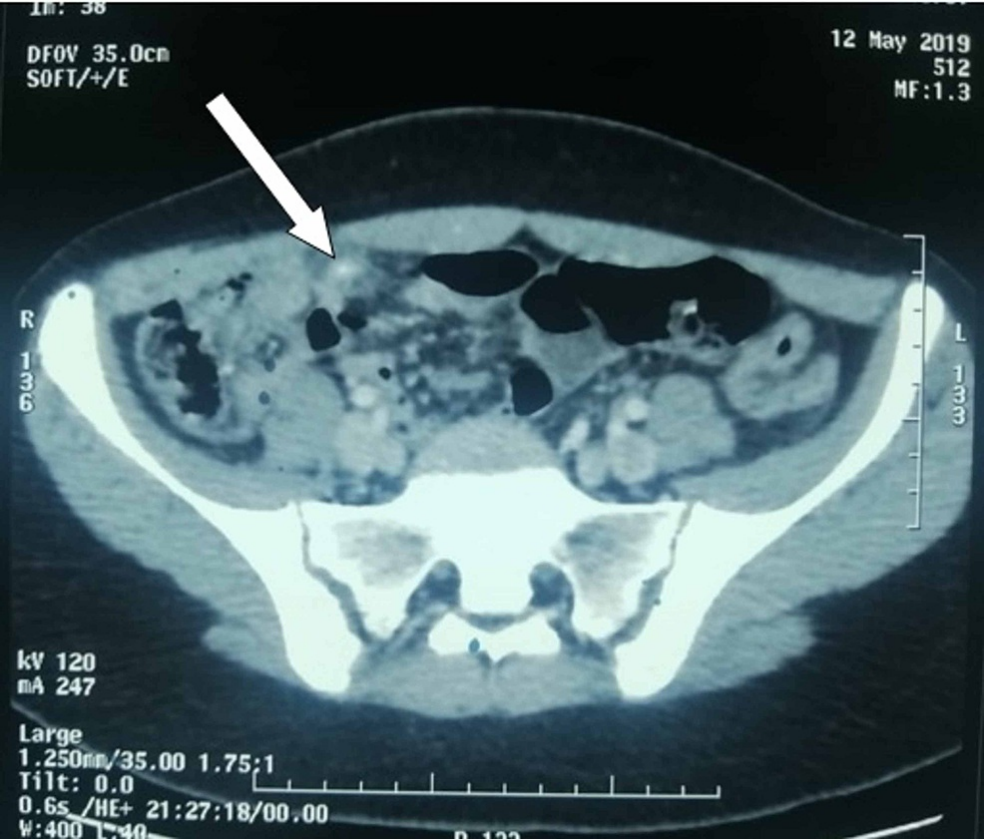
Axial contrast-enhanced CT showing of the abdomen showing dilated appendix associated with appendiceal wall thickening in favor of acute appendicitis and the presence of a linear spontaneously hyperdense image of 3 cm in length (white arrow)
